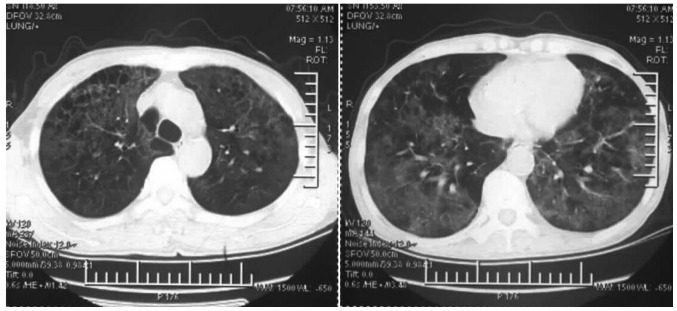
Chest computed tomography on October 19, 2007: Diffuse ground-glass shadows in the bilateral lungs and nodule shadows in the hilar region.
